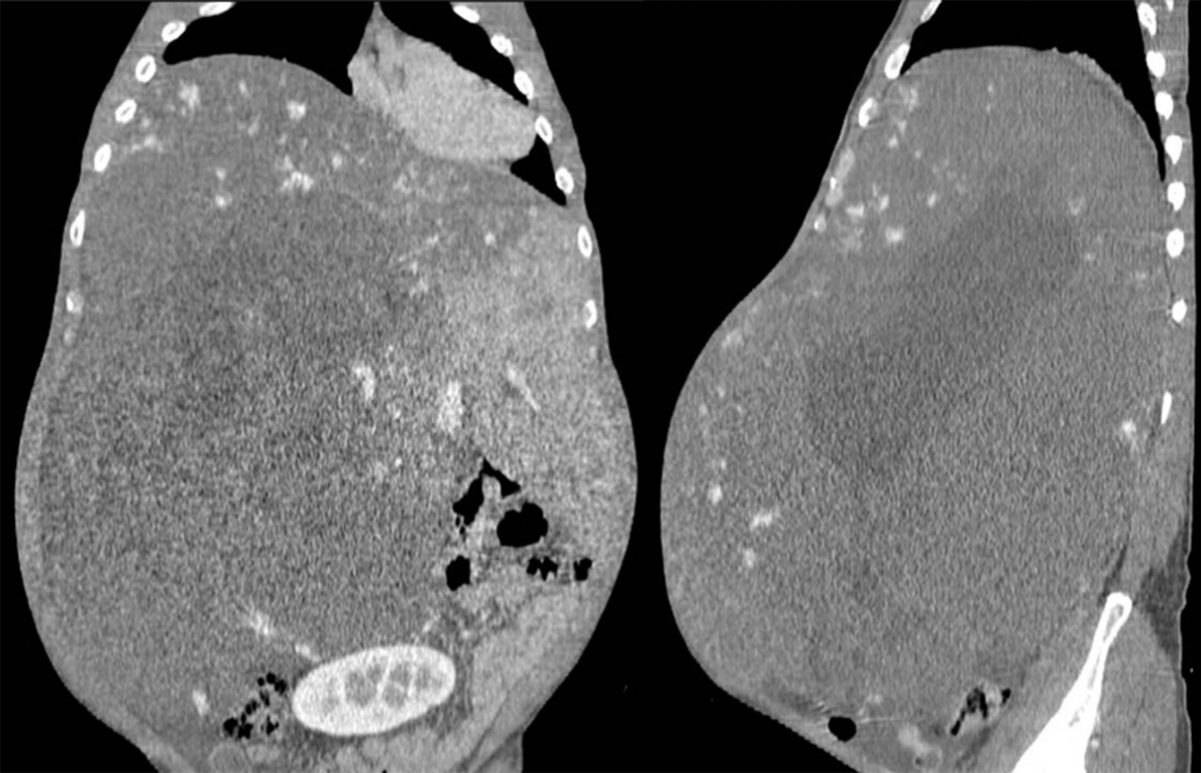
Coronal and sagittal views of the hemangioma. Reconstructed from multi-detector computed tomography images.
